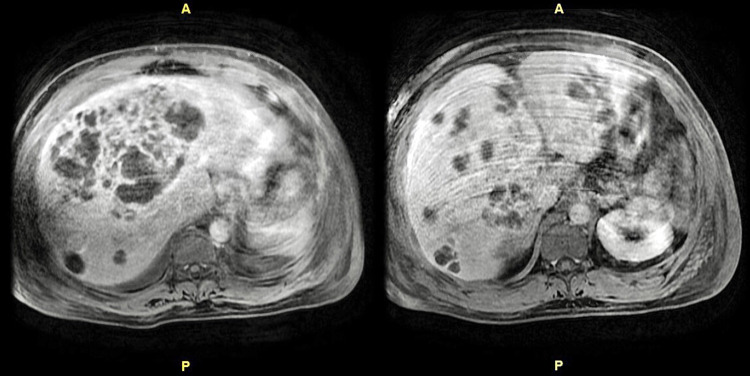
Triple-phase magnetic resonance imaging of the abdomen redemonstrating innumerable hepatic abscesses with the largest lesion demonstrating phlegmon-like features.
